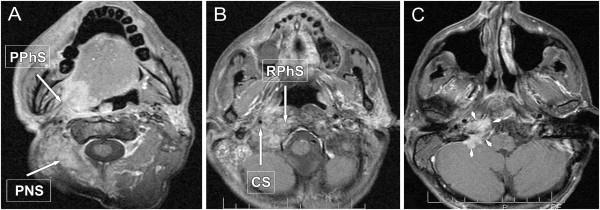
Oropharyngeal cancer, arising from the base of the tongue on the right side, stage T4b A, B, C: axial post-contrast T1-weighted MR images with fat suppression. The estimated tumour stage by clinical examination was T2, but note the tumor extension in the deep cervical structures, trough the parapharyngeal- (PPhS), carotid- (CS), retropharyngeal-(RPhS) and posterior neck space (PNS, long arrows) into the scala posterior, involving the apex of petrous part of temporal bone and pontocerebellar region (arrowheads).
